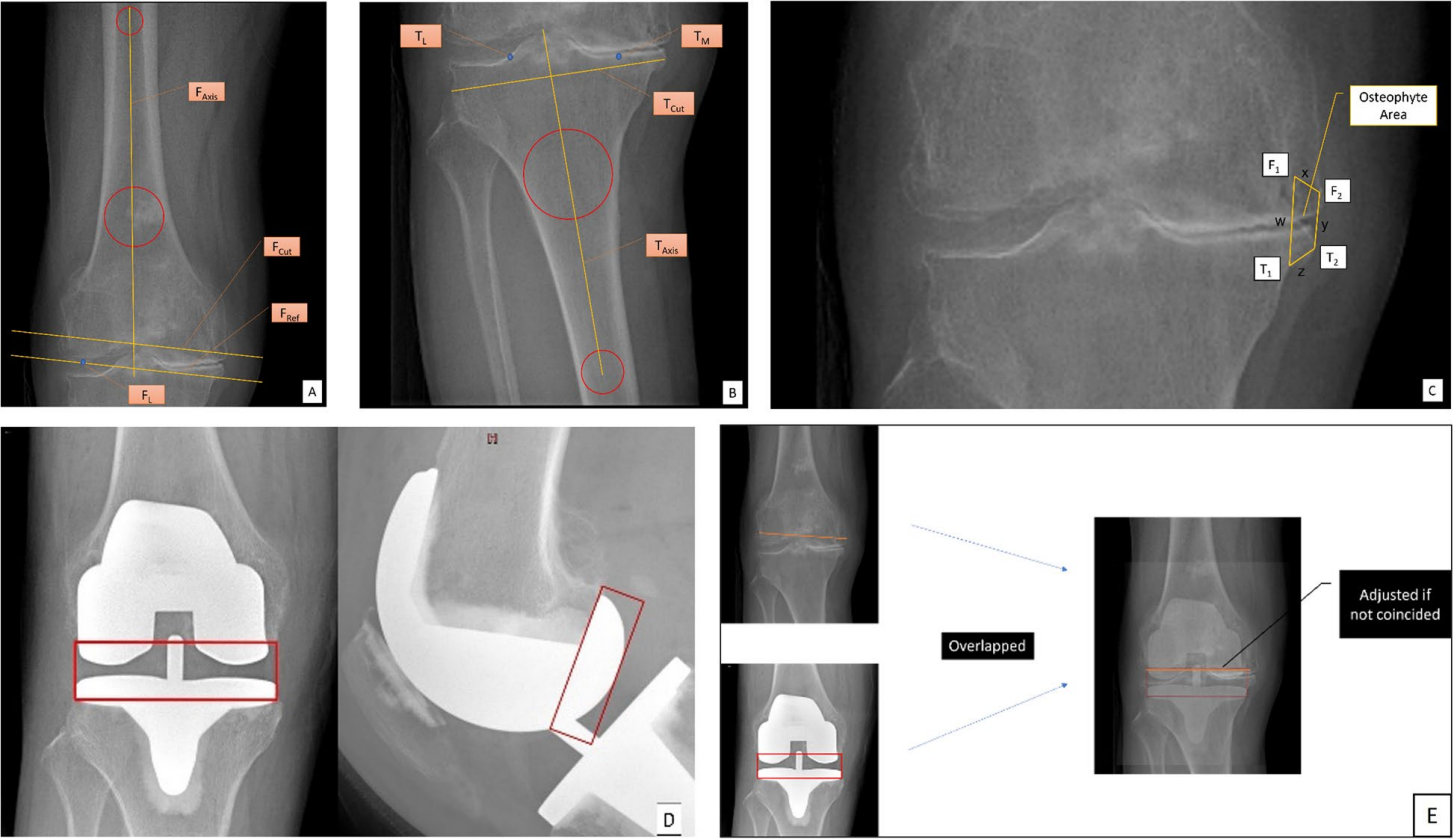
Radiographic labeling and calibration of preoperative and postoperative X-rays. Labels on AP view demonstrated elements defined in Table 1. A & B, preoperative X-ray. C, osteophyte area. D, postoperative X-ray. E, calibration of magnification
